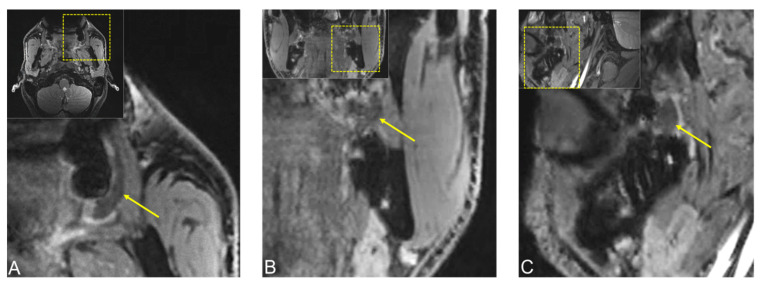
Preoperative MRI of the same lesion using 3D double-echo steady-state (3D-DESS) imaging protocol. (A) Axial, (B) coronal, and (C) sagittal reconstructions visualizing a well-demarcated lesion with a maximum extension of 21.3 × 5.6 mm (axial), 13 × 8.6 mm (coronal), and 10.1 × 9.8 mm (sagittal). For orientation, the dotted rectangles in the corner show the enlarged area. The MR image reconstructions seem to have a slightly lower resolution due to the larger scale along the slice direction.
